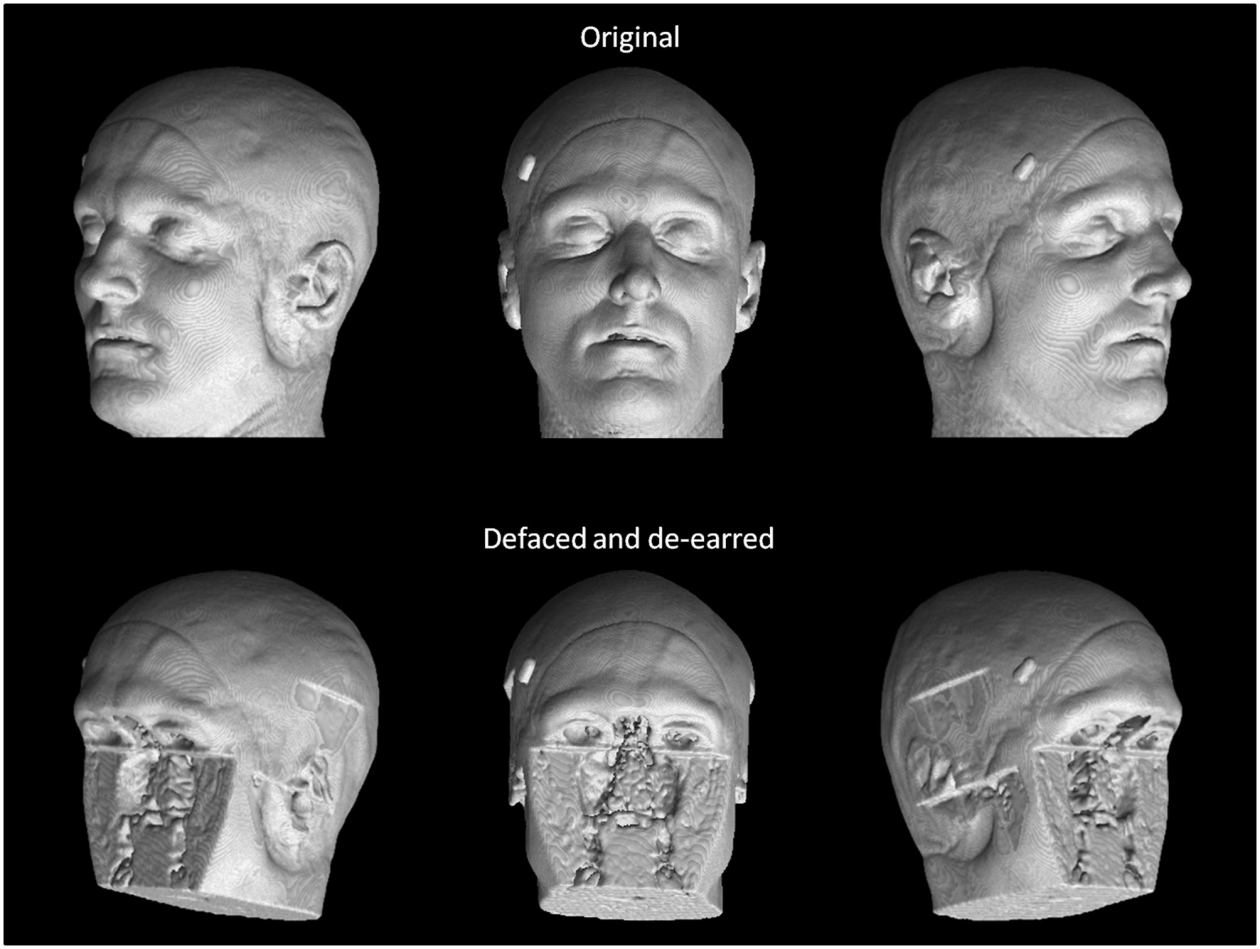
True facial recognition task images. Top row: Sample original (pre-defaced) 3D rendered T1 image. Three perspectives of the head were generated, including 45° left, straight on, and 45° right. Bottom: The same image after undergoing defacing (in this case, pydeface) and de-earring. Consent was given by the participant to include their non-defaced MRI render in the publication.
